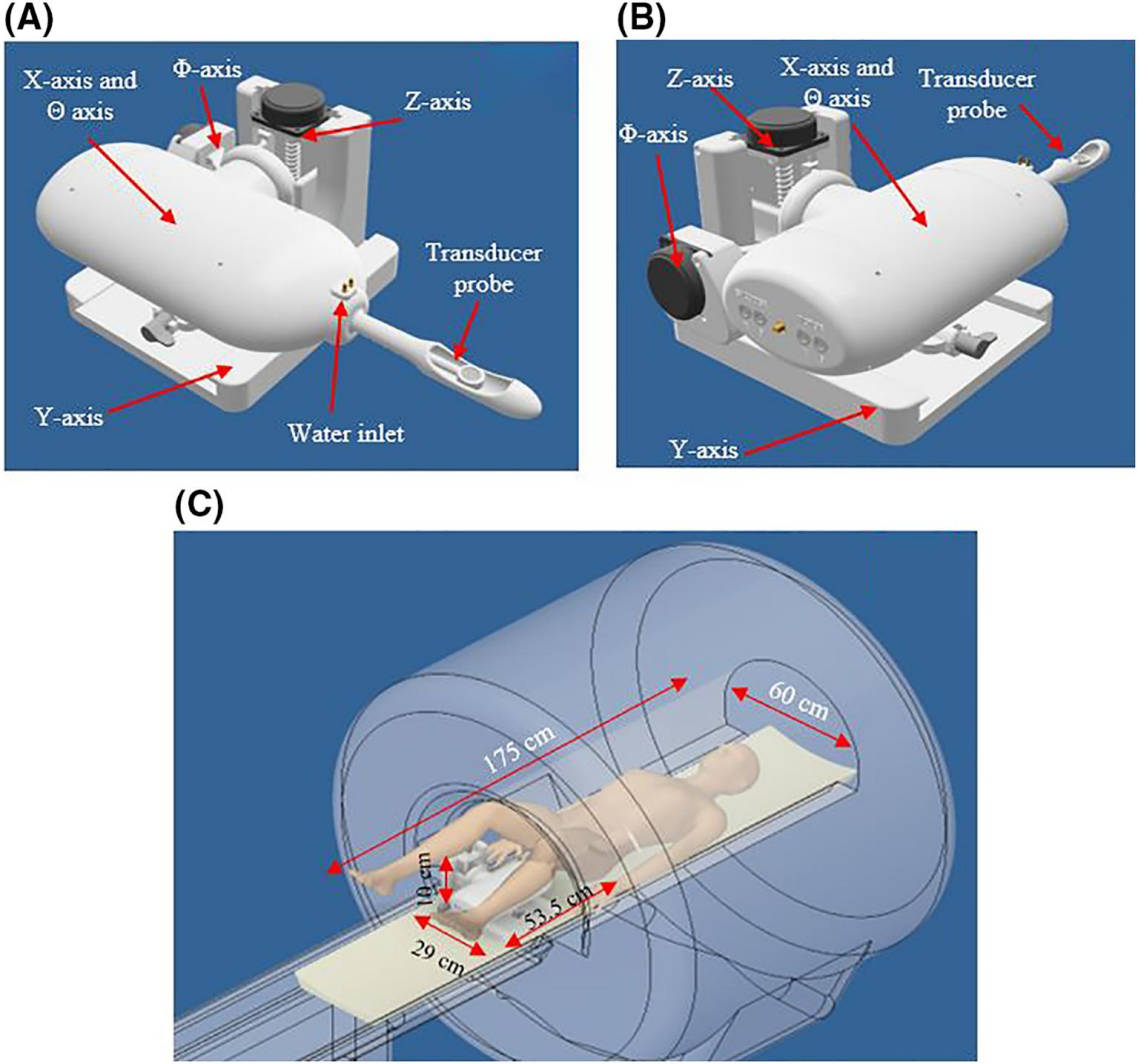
(A) Front view of the CAD design of the robotic system. (B) Rear view of the CAD design of the robotic system. (C) Introduction of the device as placed on the MRI table. CAD, computer‐aided design; MRI, magnetic resonance image
